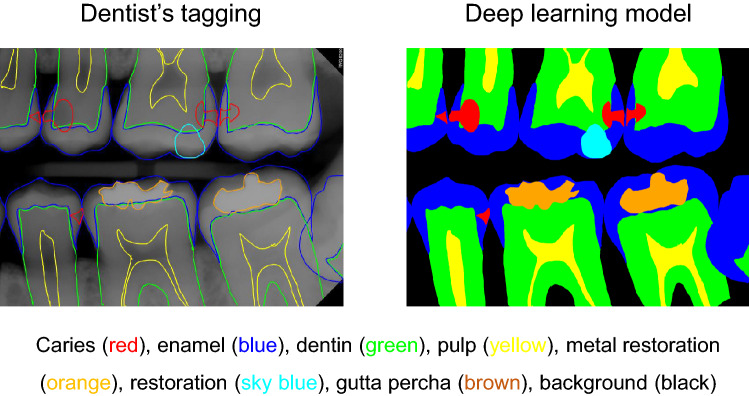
Example of the analysis of dental structures and caries tagging. The observers drew lines for the segmentation of dental structures (enamel, dentin, pulp, metal restoration, tooth-color restorations, gutta percha) and dental caries on the bitewing radiographs. *No software was used to generate the image. The picture used in Figure is a file printed using the source code that we implemented ourselves.
